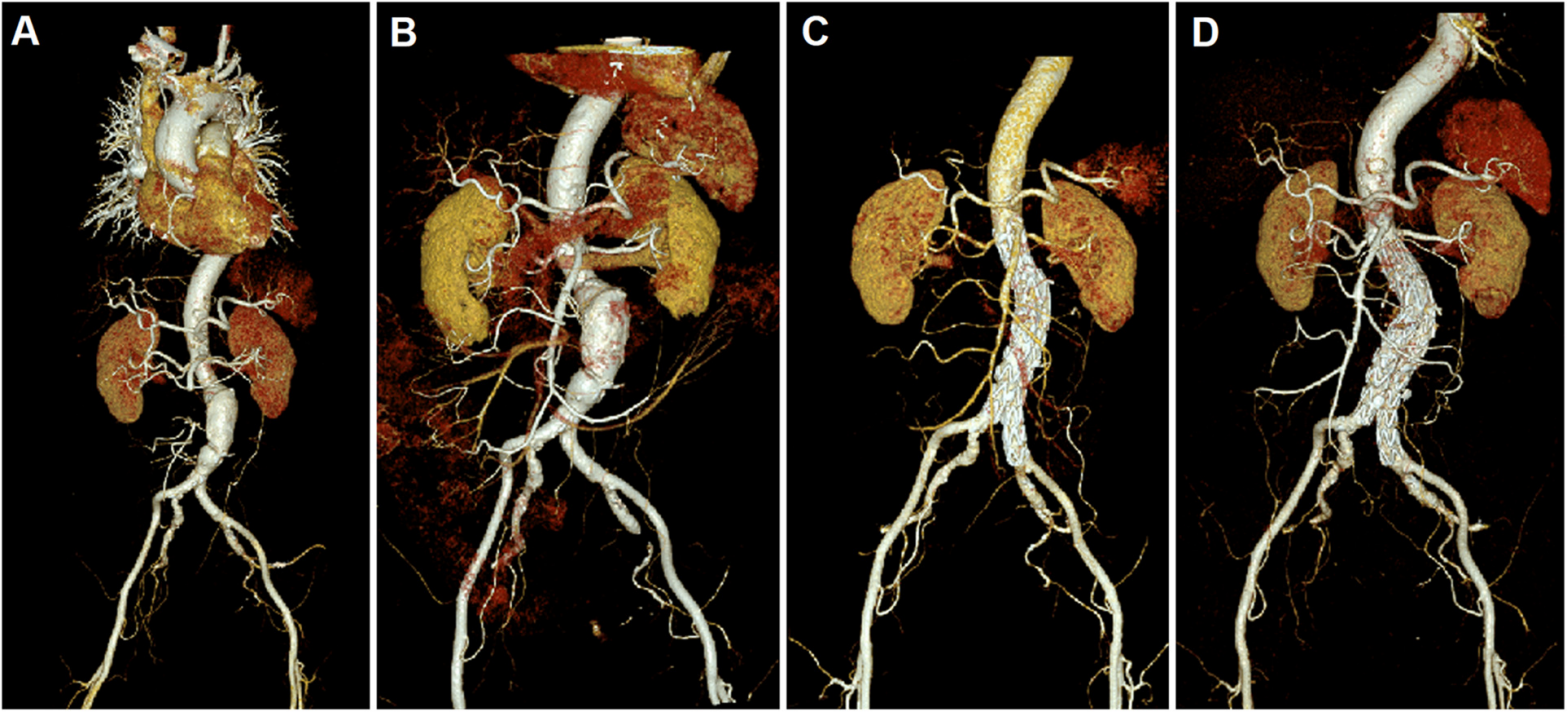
CTA figures before and after surgery. (A) Before surgery, an abdominal CTA indicated the presence of an ASMAE and an AAA. The larger cross-section of AAA was measured approximately 5.4 * 4.9 cm, with an involved length of about 8.8 cm; (B) A CTA performed on the 12th day after ASMAE treatment confirmed the patency of the main trunk and distal branches of the SMA; Follow-up CTA at 6 months (C) and 38 months (D) indicated that the SMA was patent, with no filling defects observed; the abdominal aortic covered stent was in place, with no significant endoleak or thrombosis.
